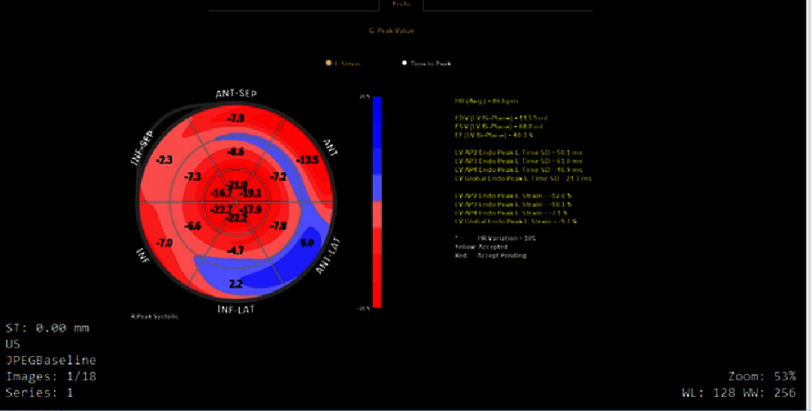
Speckle-tracking echocardiographic global longitudinal strain (GLS) bull’s-eye plot demonstrating severely reduced myocardial strain with a calculated GLS of −9.7%. The segmental strain map shows marked reduction in basal and mid-ventricular longitudinal deformation with relative preservation of apical strain, producing the characteristic apical sparing (“cherry-on-top”) pattern that is highly suggestive of cardiac amyloidosis in the appropriate clinical setting.
